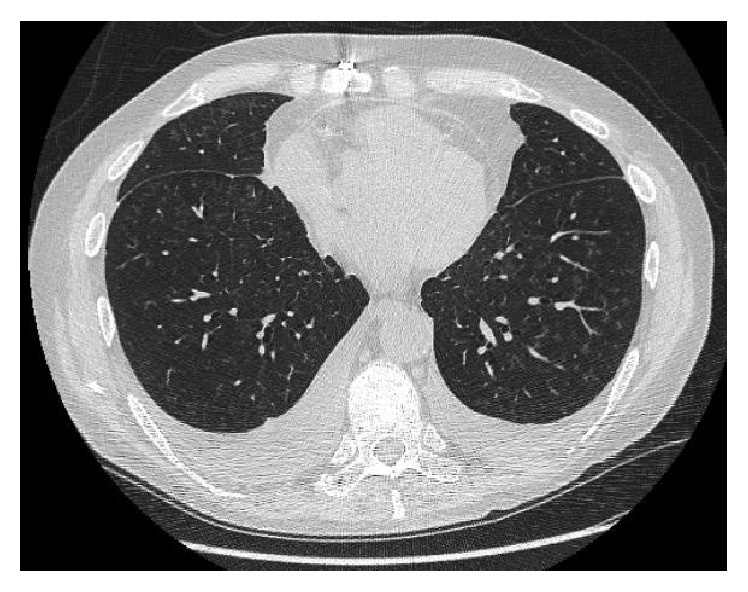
High-resolution axial computed tomography scan of the chest shows ground-glass centrilobular nodules before initiation of everolimus therapy.
